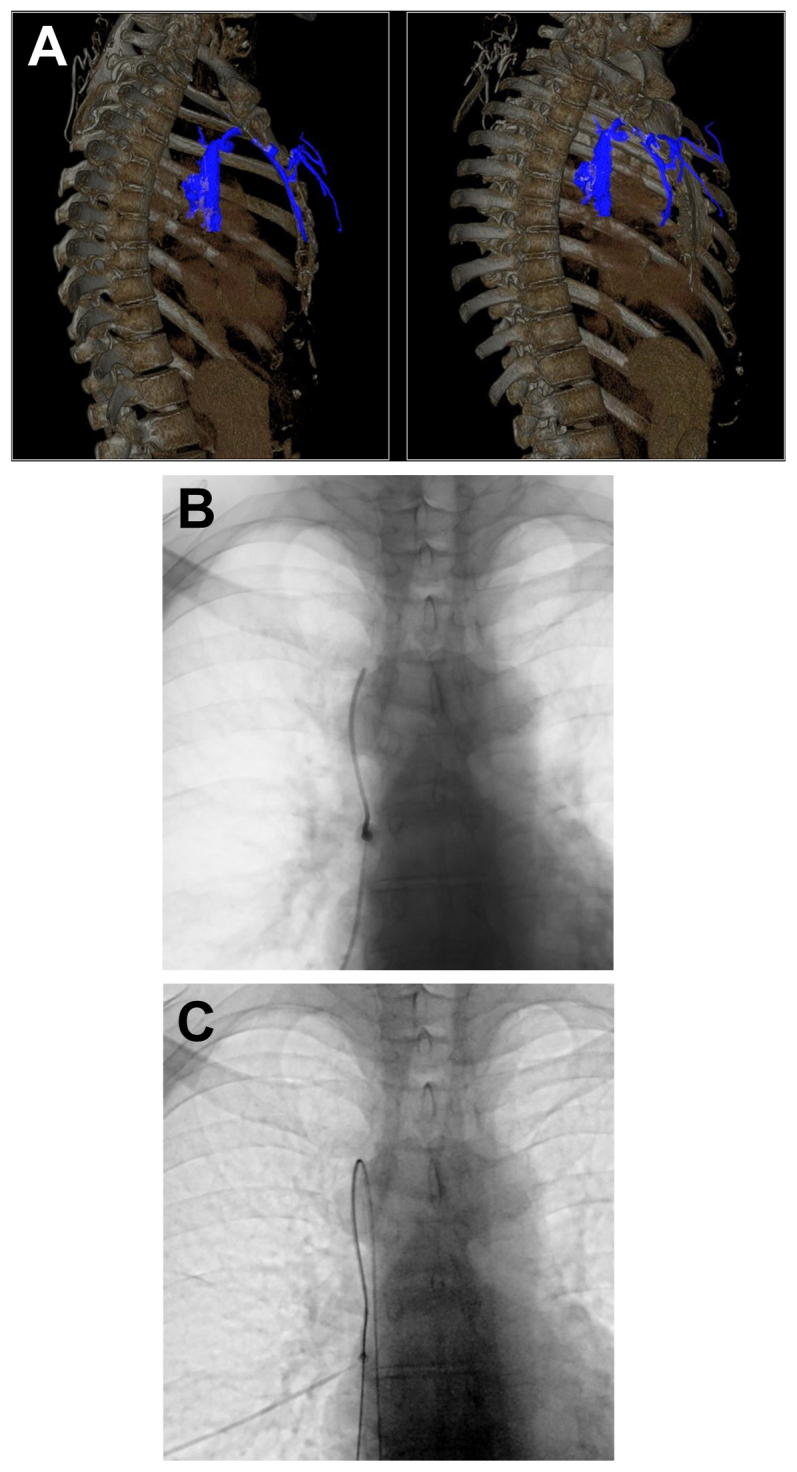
The initial computed tomography venography revealed chronic occlusions of the bilateral internal jugular and subclavian veins, which were caused by catheter-related thrombosis. Additionally, a large right internal thoracic vein with a diameter of 4 mm was detected (*A*/cover).
